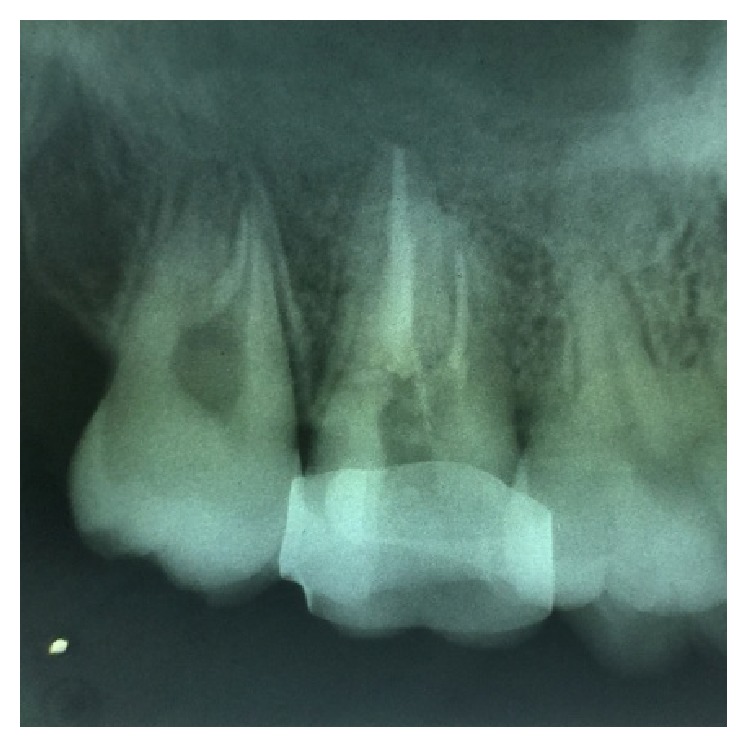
Radiograph showing 3 mm of intracanal space preparation with respect to 27.
